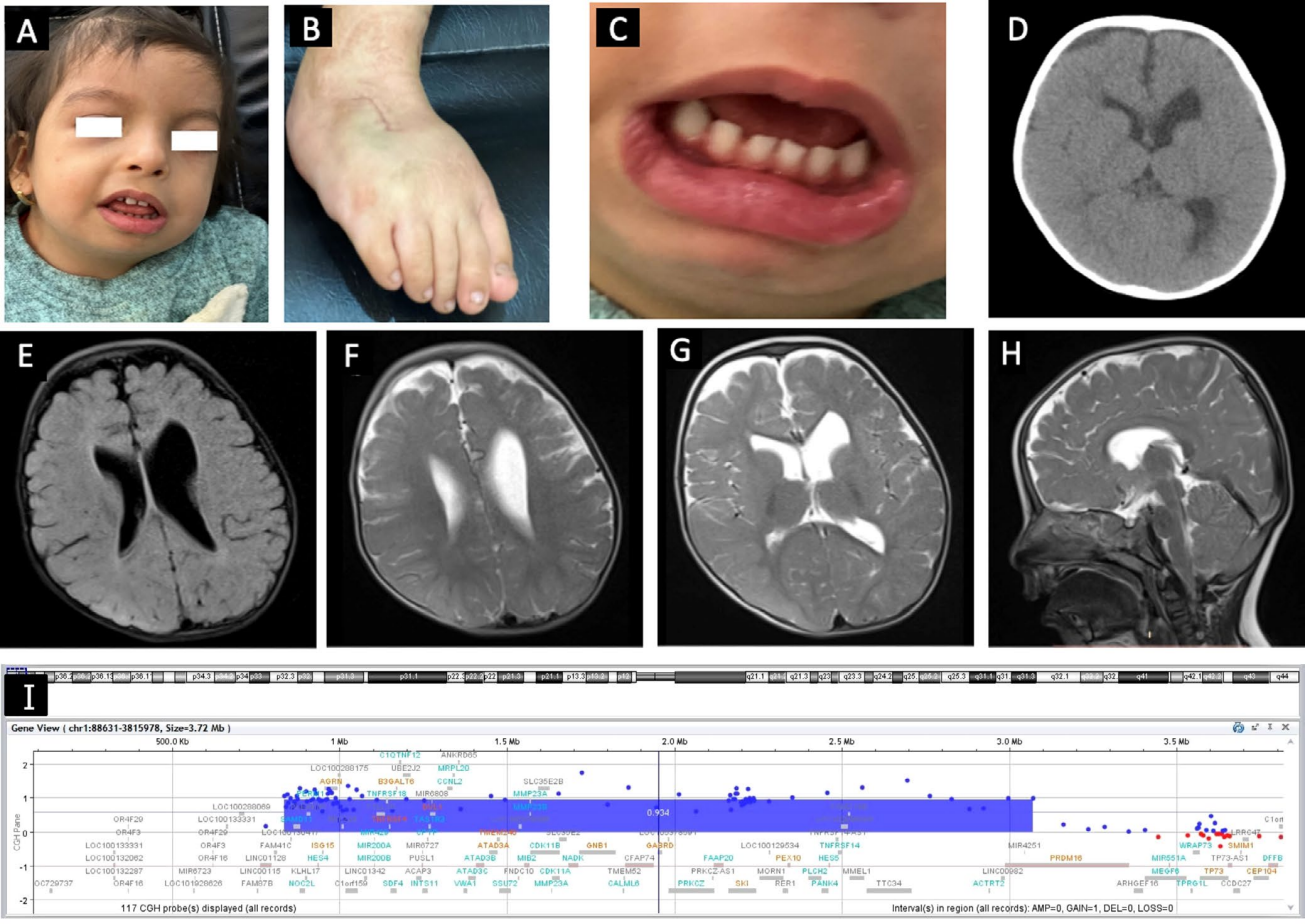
Clinical photographs, brain MRI and CT scan, and array‐CGH results of the patient with 1p36.33 duplication. Clinical photographs showing facial dysmorphism (A), surgically corrected right clubfoot (B), and widely spaced teeth (C). The axial brain CT scan shows left‐side ventriculomegaly without any calcification (D), and the T1 (E) and T2 (F, G, H) sequences of brain MRI show ventriculomegaly especially on the left side with a mild abnormality of cortical gyration on the frontal region. Array‐CGH results indicate a duplication at the 1p36.33p36.32 (GRCh37/hg19; chr1:834,101–3,070,509) (I); genomic coordinates elsewhere in the manuscript are reported on (GRCh38/hg38).
